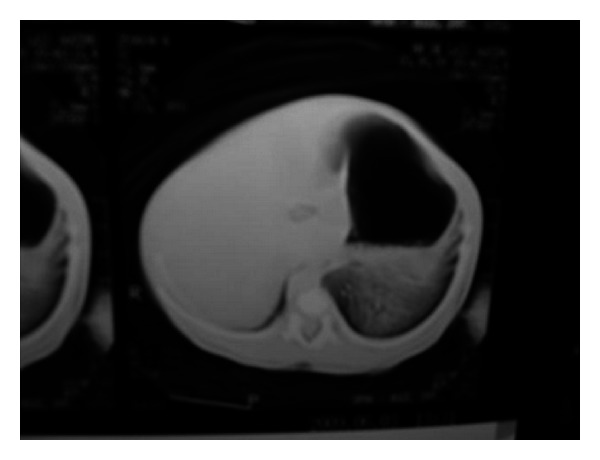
Postnatal computed tomography in axial plane showing a great cyst image in left hemithorax compression of the normal left lung tissue and contralateral mediastinal shift.
